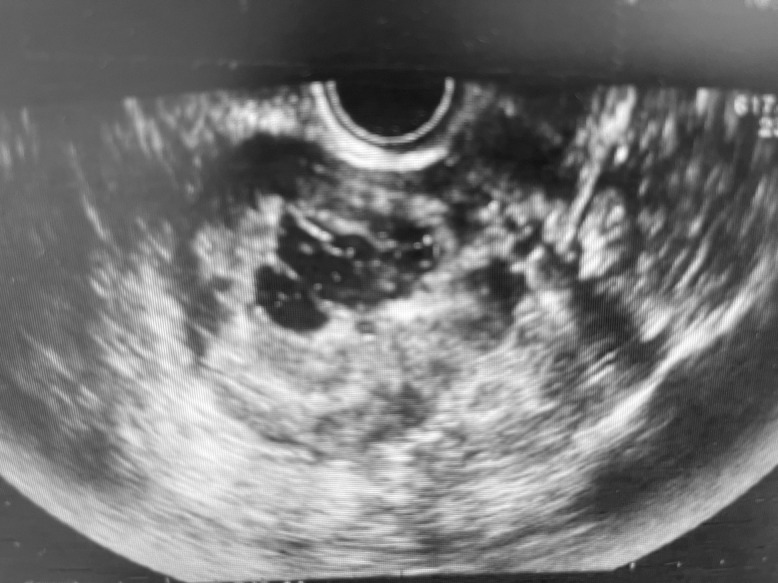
Ultrasound image obtained 7 days postoperatively showing a cystic lesion in the uterine cavity.
